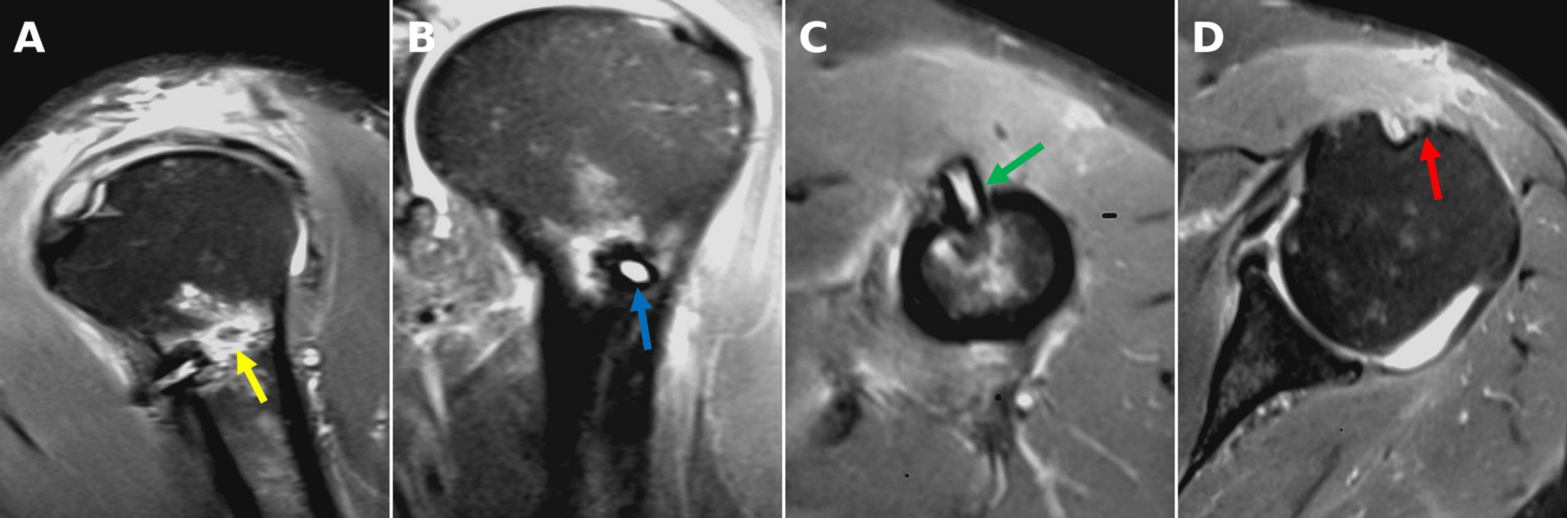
Eight weeks postoperative T2-weighted MRI of the left shoulder. (A) Screw cut-out with intraosseous inflammation (yellow arrow). (B) Sagittal view of screw penetration through proximal humerus (blue arrow). (C) Axial view of screw penetration through proximal humerus (green arrow). (D) Empty bicipital groove (red arrow). MRI: magnetic resonance imaging.
